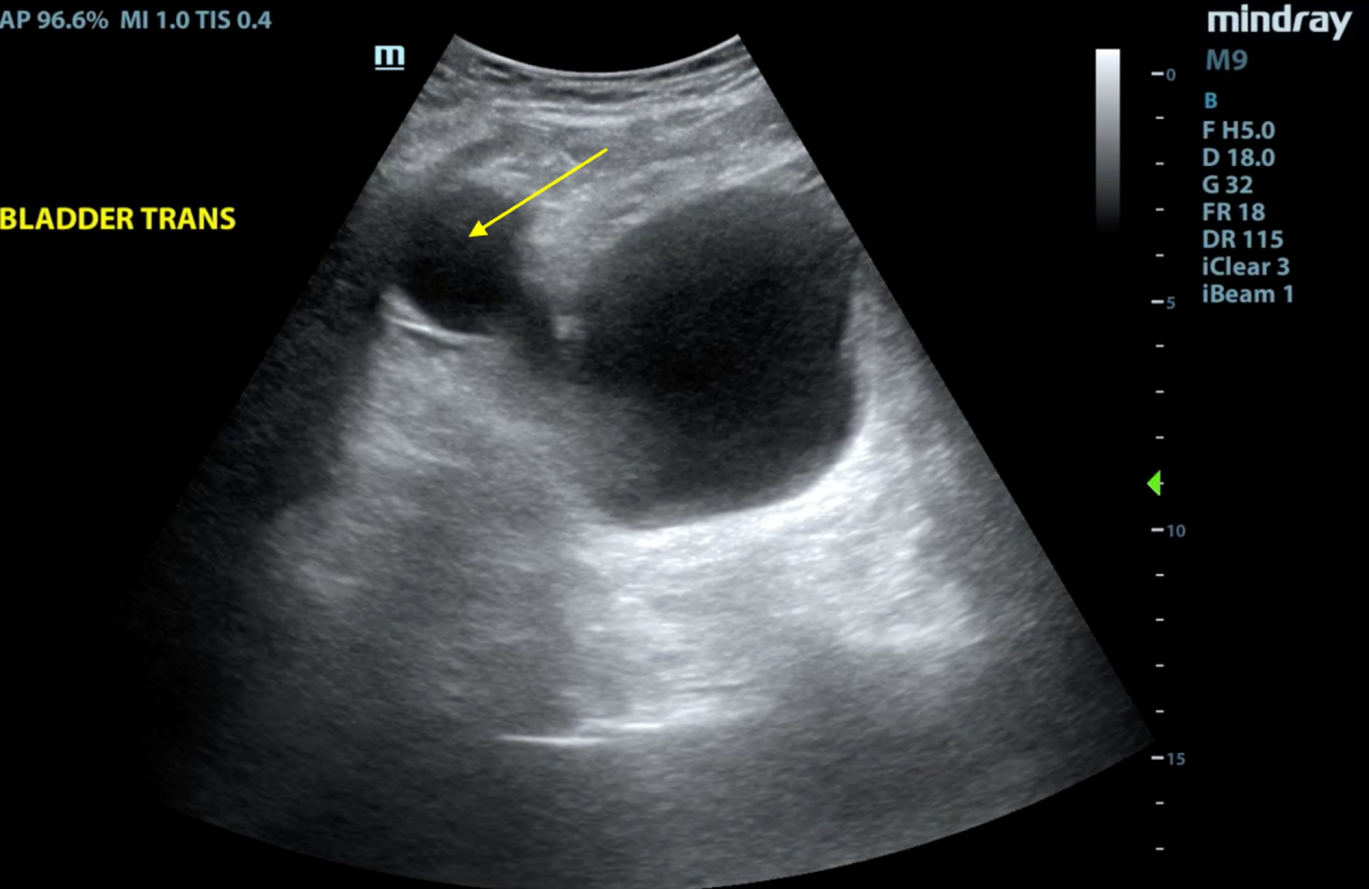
Bladder ultrasound image The bladder ultrasound image in the transverse view prior to voiding shows a right-sided “cystic mass” that is consistent with the normal positioning of the balloon of the artificial urinary sphincter at the right iliac fossa, with appropriate appearance (marked by the arrow).
